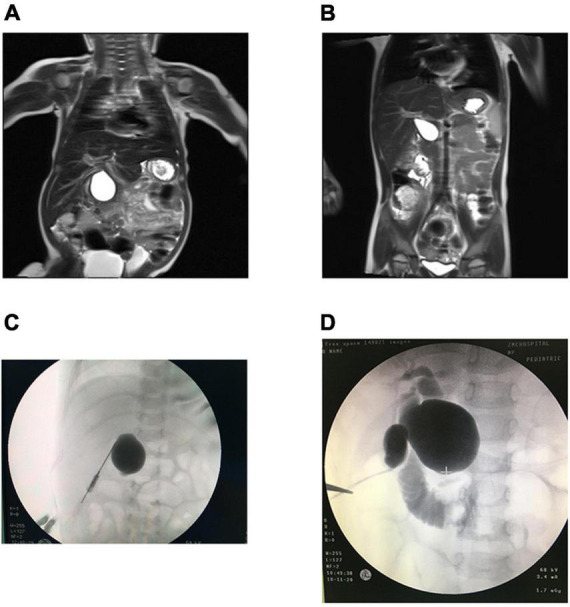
MRI in CBA and CC patients. (A) CBA: hepatic hilar cyst. (B) CC: well-circumscribed, preportal cyst. IOC in CBA and CC patients. (C) CBA: only cysts were visualized. (D) CC: the gallbladder, cyst and duodenum were visualized.
